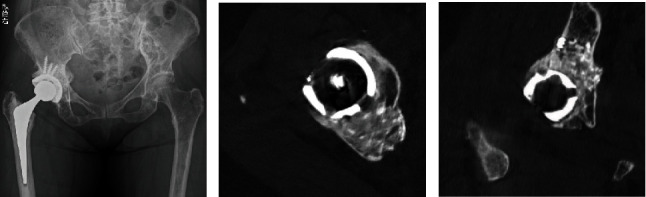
(a) Plain radiograph and CT images (b) axial and (c) coronal at 1 year after operation reveal incorporation of the acetabular bone grafts.
